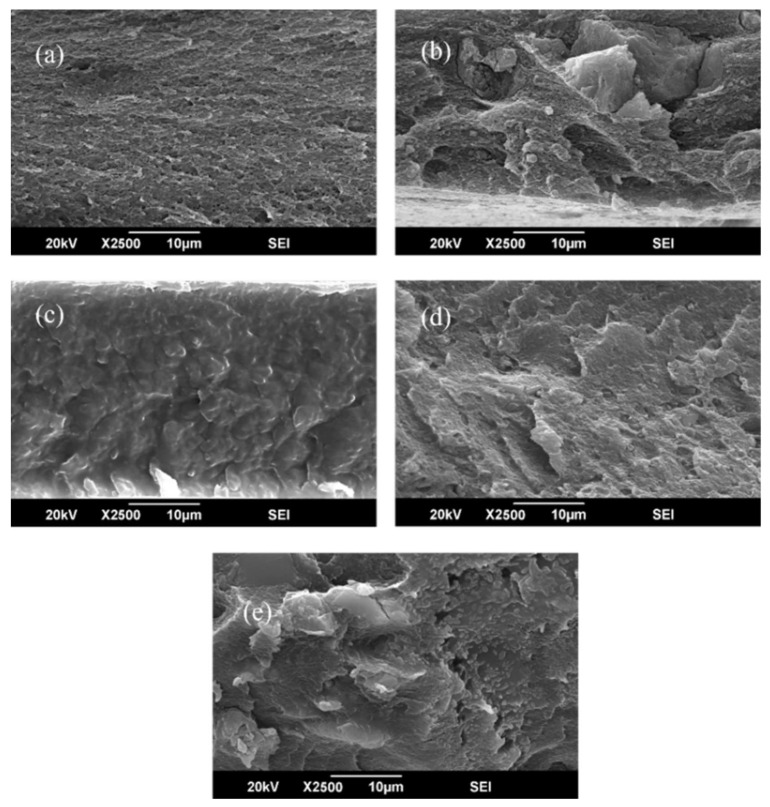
Cross-sectional morphology of membranes: (a) pristine CTA, (b) CTA/Cu-BTC-5 wt.%, (c) CTA/Cu-BTC-10 wt.%, (d) CTA/Ni-Cu-BTC-10 wt.%, and (e) CTA/Cu-BTC-15 wt.%.
